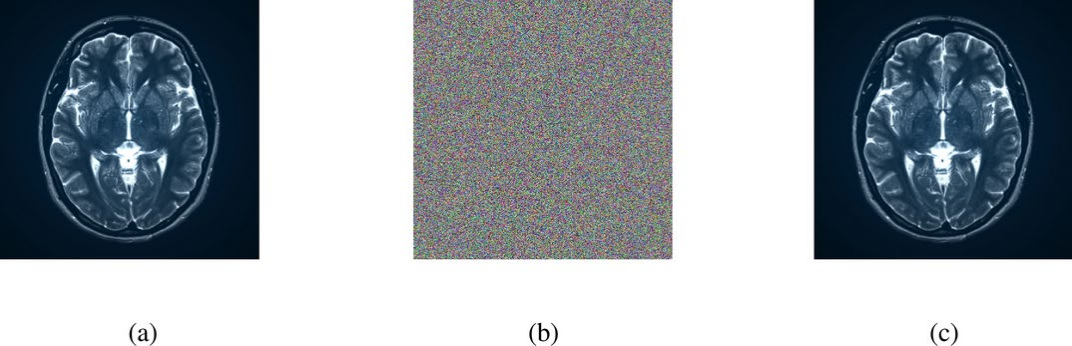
(a) Original brain MRI image, (b) Decrypted image with a small change in the initial condition of $$K_4$$, (c) Correctly decrypted image.
